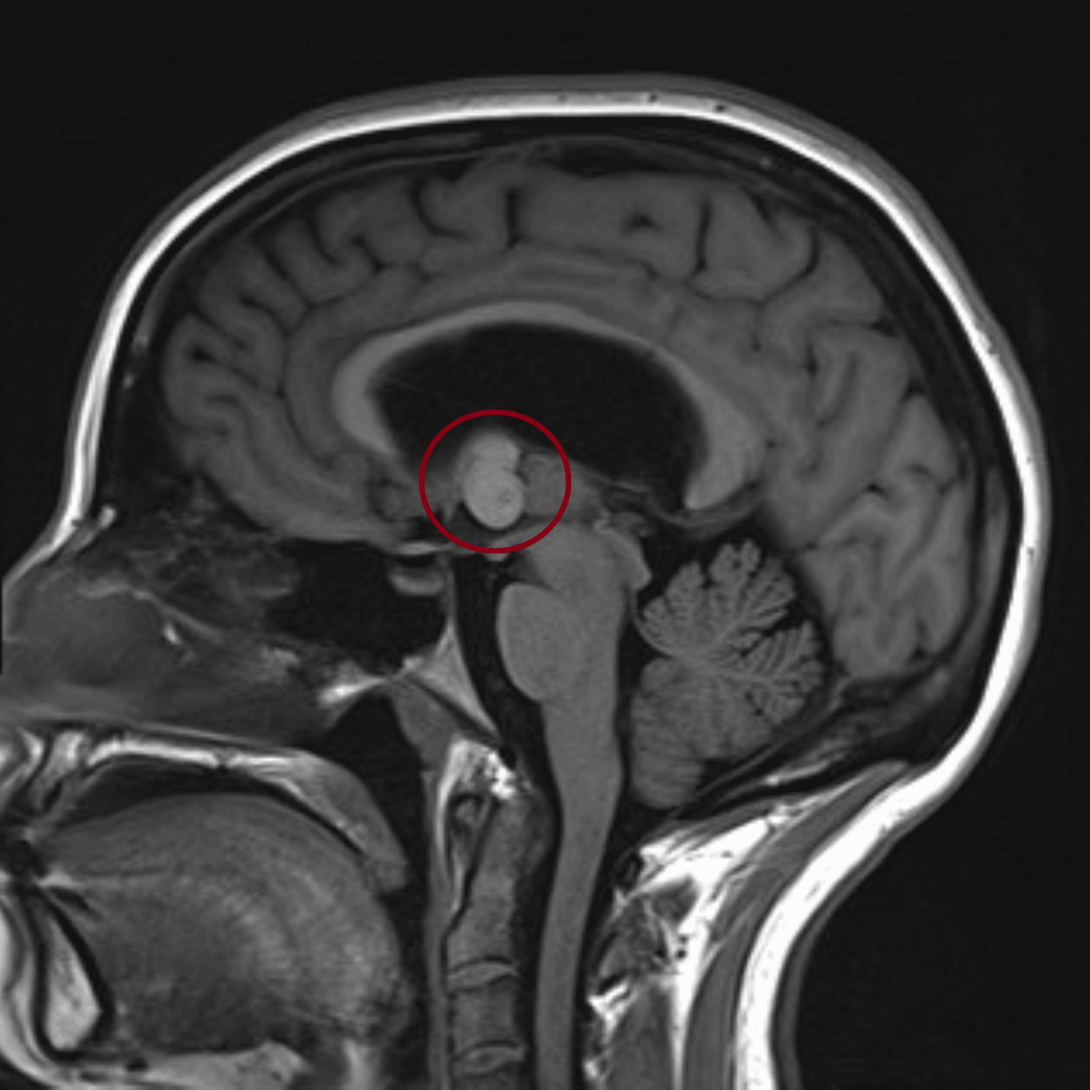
Sagittal T1-weighted MRI demonstrating a hyperintense, well-defined colloid cyst at the foramen of Monro with associated ventricular dilation.
